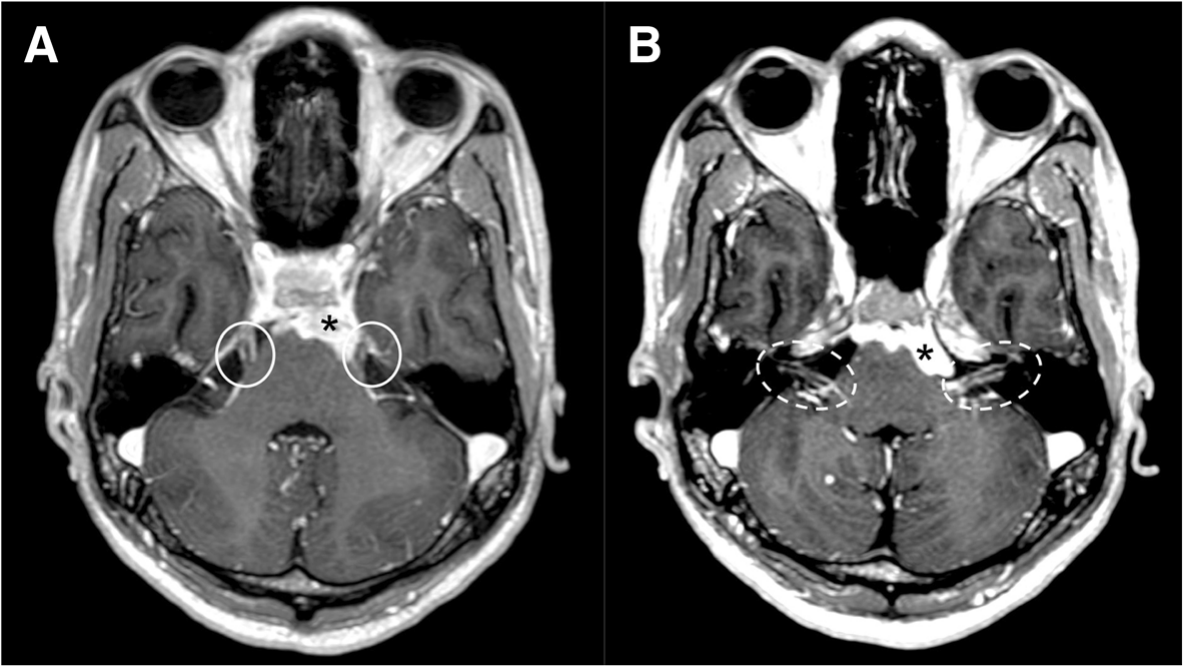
Tuberculosis (TBC). MRI T1-weighted post-gadolinium axial image demonstrates pathologic enhancing tissue involving the basal cistern and ventral surface of the brainstem (asterisks), with leptomeningeal enhancement of bilateral trigeminal (a, circles), facial, and vestibulocochlear nerves (b, dotted circles)
